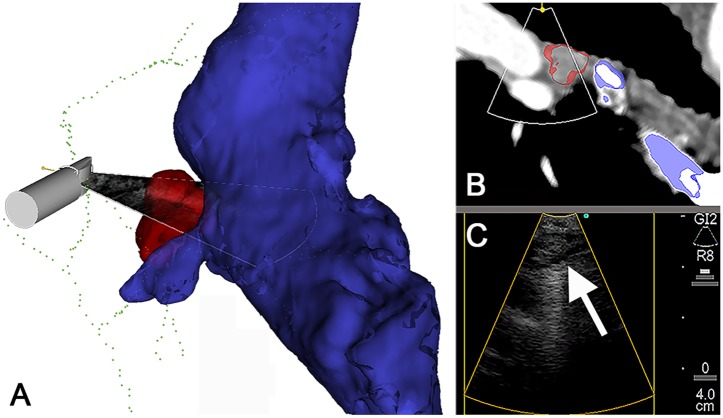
Navigated EBUS-TBNA used to assess operability in patient 3. (A) Preoperative CT indicated a non-curative setting due to a probable lymph node metastasis proximal to the azygos vein (blue). Intraoperative multimodal images correctly placed the lymph node (red) to a different level than CT, concluding with less advanced disease. For improved overview of the vasculature, the airway CT models were not included in this visualization. (C) Real-time EBUS imaging the azygos vein and 10R lymph node (arrow). (B) Corresponding CT plane with US sector position indicated (overlaid the CT).
